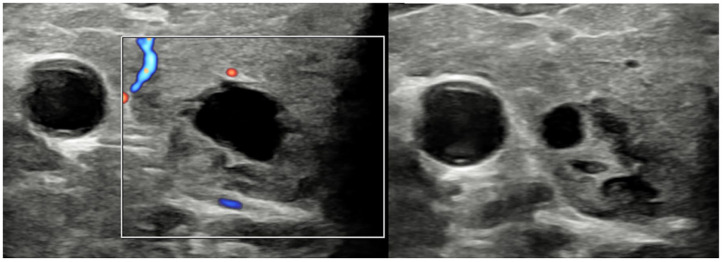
Both thyroid lobes contain multiple well-defined, mixed cystic and solid nodules with no internal Doppler vascularity. These were described as cystic or spongiform – U2 nodules initial visit.
